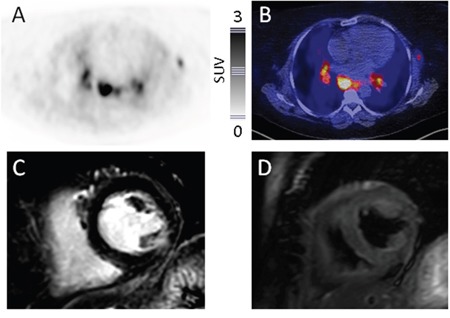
Display of a patient without myocardial sarcoidosis Consistency of ^68^Ga-DOTATOC-PET and CMR Display of transaxial ^68^Ga-DOTATOC-PET (A., color bar indicating standardized uptake values), fused PET/CT B., short-axis late gadolinium enhanced (LGE) C., and short-axis T2-weighted CMR D. slices in a 51-year-old woman (patient #6) with multi-organ sarcoidosis. PET presents enhanced tracer uptake in a left axillary and mediastinal lymph nodes, highly consistent with active sarcoidosis. In correspondence to CMR, no signs of myocardial involvement can be recorded. Follow-up is unremarkable regarding cardiac events.
